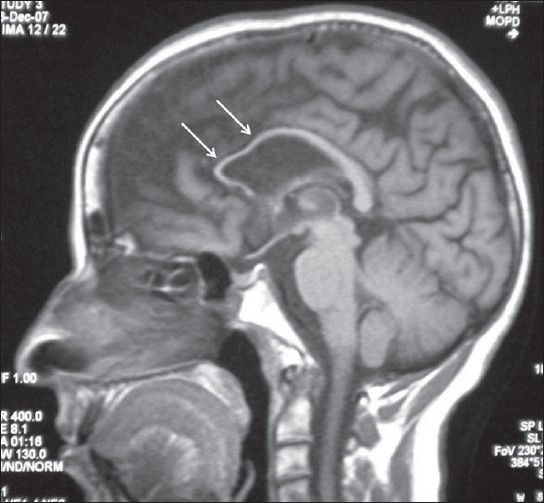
Mid-sagittal T1-weighted MR image showing thin corpus callosum mainly involving rostrum and genu (arrows) with frontoparietal lobe atrophy
